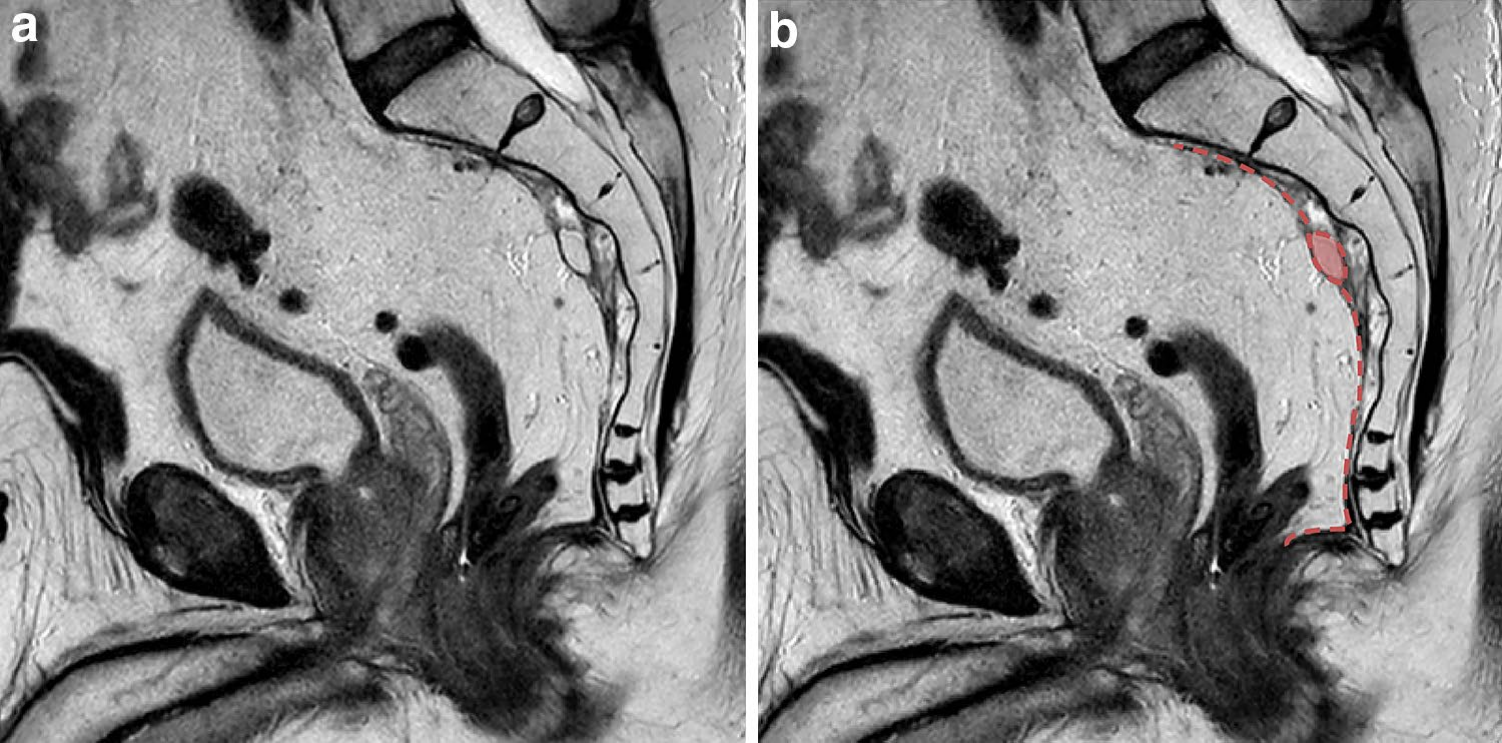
MRI-detected residual mesorectum following transanal total mesorectal excision (TaTME). a, b Sagittal T2-weighted MR-images showing cranially located residual mesorectum in relation to the anastomosis. b Residual mesorectum coloured in red. Red dashed line showing the level of dissection and anastomosis after TaTME. (Color figure online)
